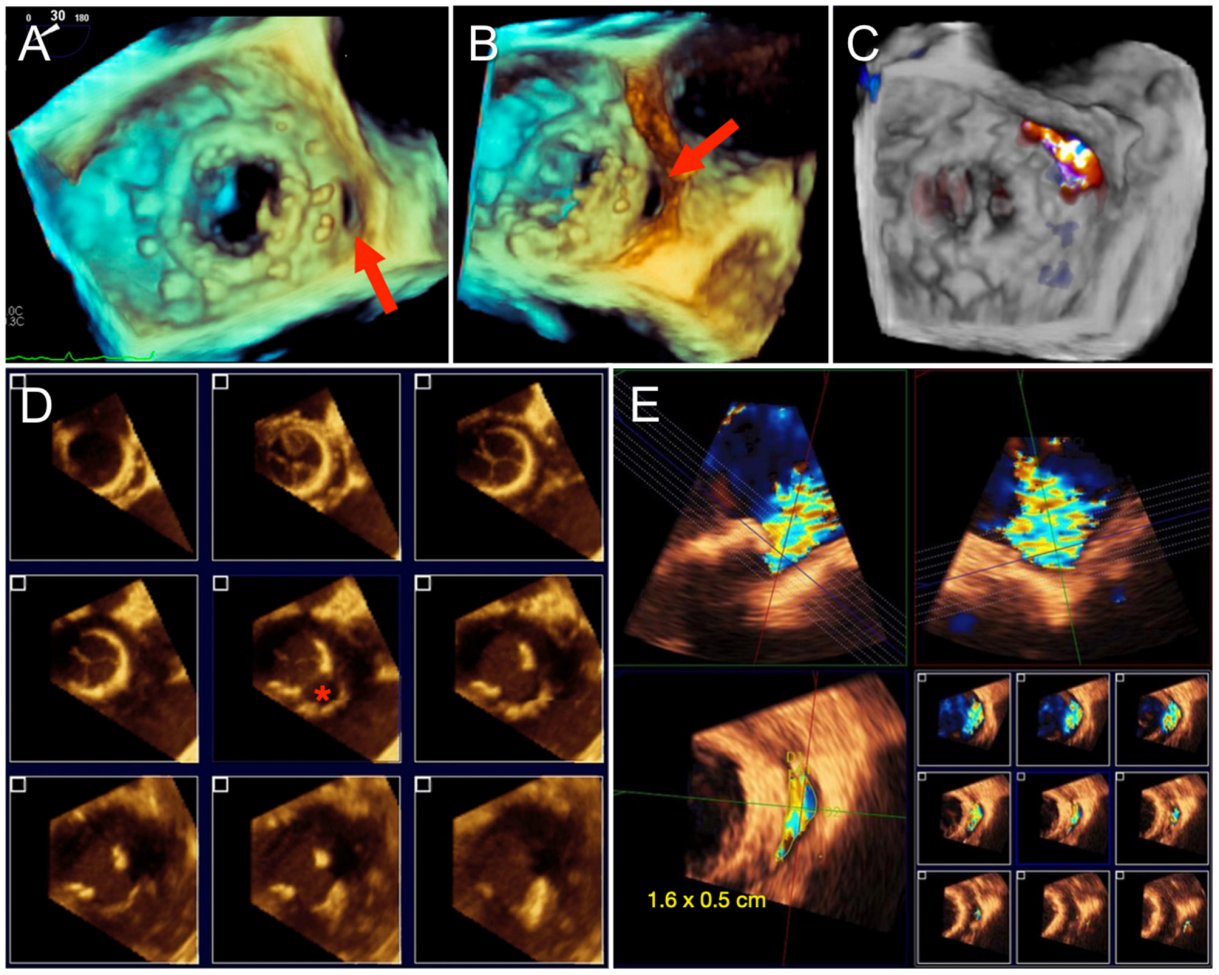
Paravalvular mitral regurgitation assessment by TEE. (A) 3D *en face* “surgeon's view” of a mitral bioprosthesis demonstrates a gap along the posteromedial aspect, corresponding to a paravalvular defect. (B) Rotation of the 3D volume improves visualization of the paravalvular defect. (C) Color Doppler confirms regurgitant flow through the defect and no other areas of paravalvular leak (PVL). Sizing of PVL by TEE can be difficult due to irregularity in the shape of the defect and dropout artifact, as shown in (D), and is best measured with color Doppler to delineate the regurgitant PVL jet and avoid overestimation (E).
